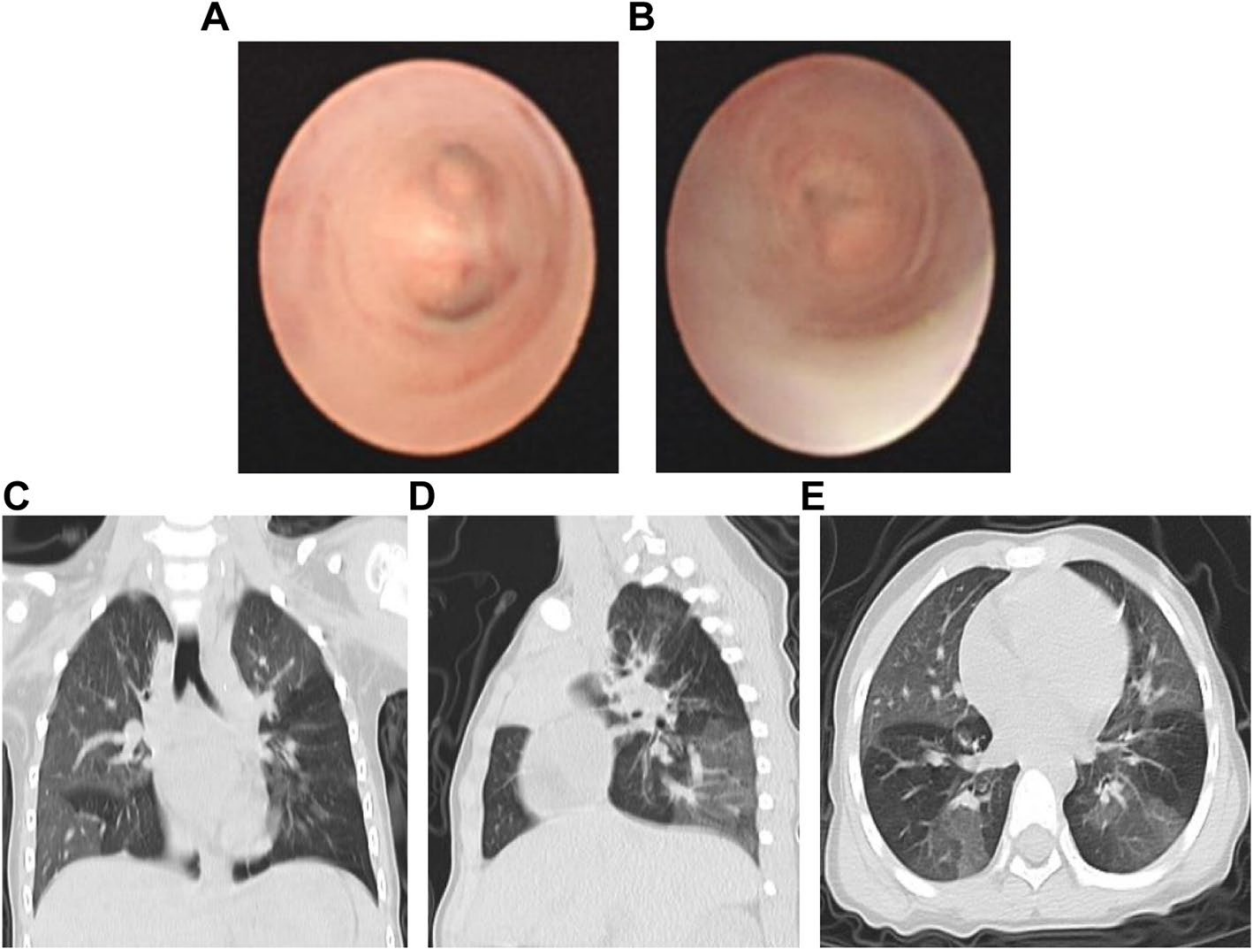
The bronchoscopic and imaging features of a child with ADVP after IMV who developed PIBO 2 years later. A-B Different degrees of middle and small bronchial lumen occlusion and fibrous plate hyperplasia were observed by using fiberoptic bronchoscopy; C-D chest radiographs of the patient from the posterior and lateral views showed that permeability of both lungs was enhanced; E mosaic perfusion sign was observed by using a CT slice
